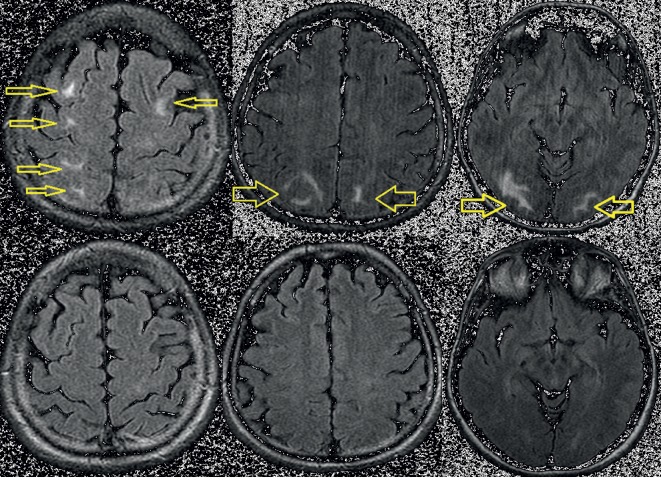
Axial FLAIR: bilateral hyperintense zones in frontal, occipital, and parietal regions. Comparative pictures (lower) showing total disappearance of lesions after 2 months (resolving vasogenic edema).
